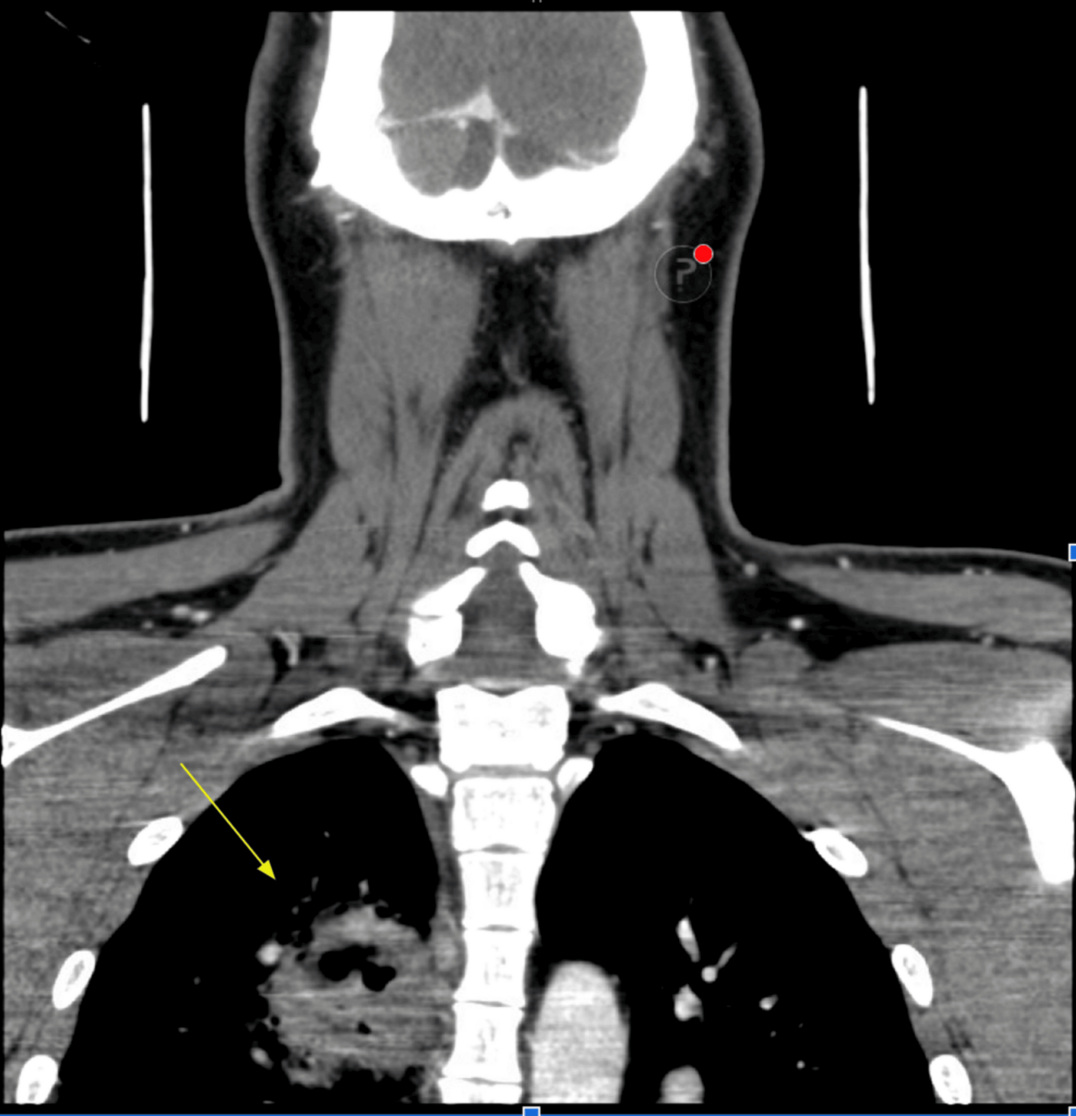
CT scan of the soft tissue neck with contrast CT scan of the soft tissue neck with contrast showing a thick-walled cavitary lesion measuring approximately 6 x 5.5 x 4.4 cm in the posterior inferior aspect of the right upper lobe. Cavitary is air-filled. No definite communication with the bronchi is identified. CT, computed tomography
